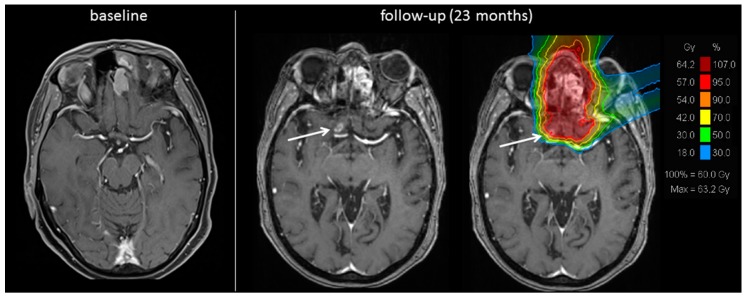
Radiation-induced cerebral contrast agent enhancement. Baseline and follow-up MRI scan of a patient with esthesioneuroblastoma (ENB). 23 months after radiotherapy a new cerebral contrast agent enhancement (white arrow) could be detected. In comparison with the initially treated radiation plan (MRI scan on the right) the lesion occurred in an irradiated region of the right frontal lobe at the edge of the 95%-isodose representing 57 Gy (RBE, 71.25 Gy EQD2). A radiation-induced disturbance of the blood-brain barrier or even radiation necrosis is probable.
